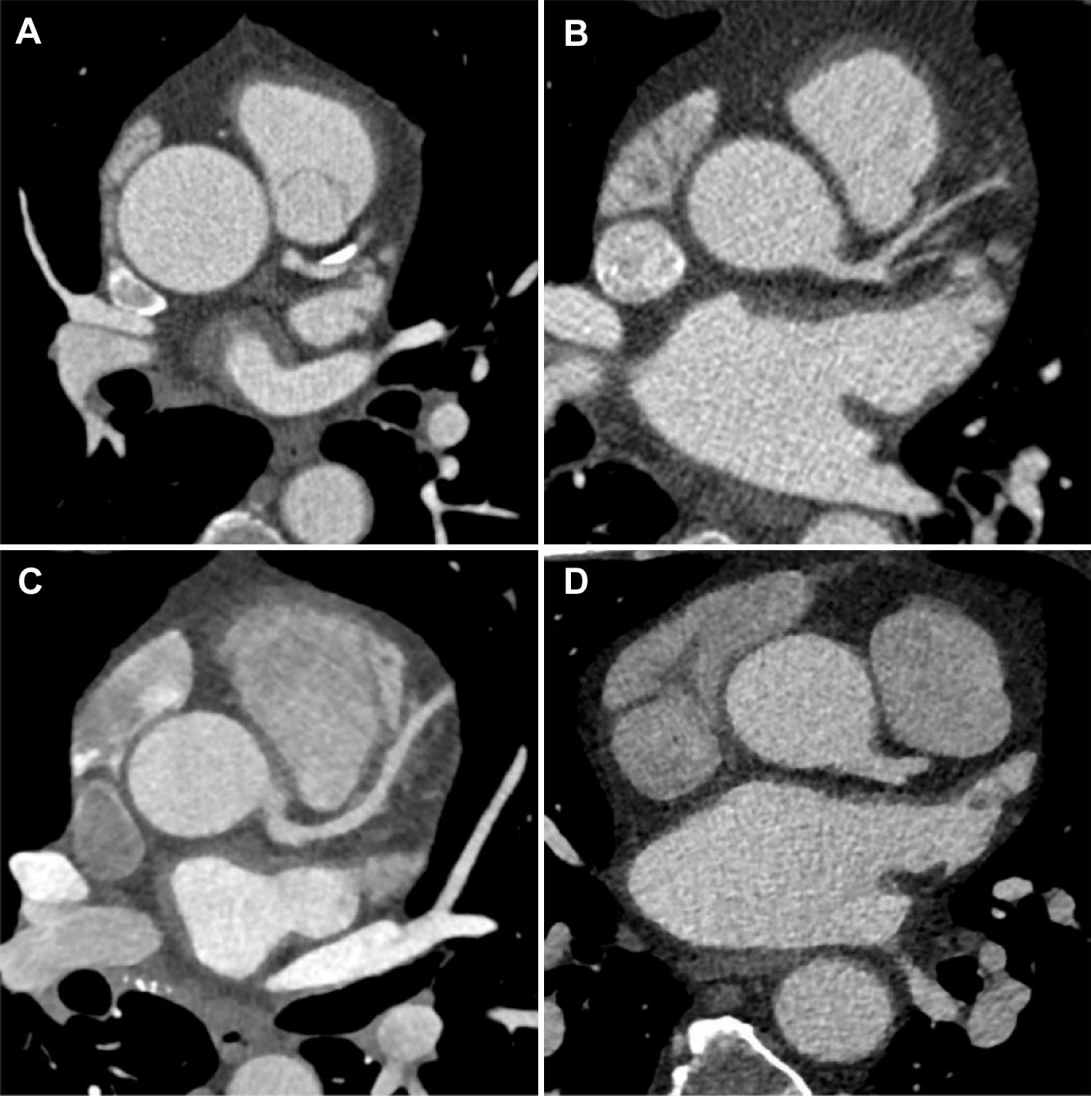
CCTA images of 4 different CT generations used for acquisition throughout the study period. Image A: 64-slice single source CT; Image B: 64-slice dual source CT; Image C: 128-slice dual source CT; Image D: 128-slice dual source CT
